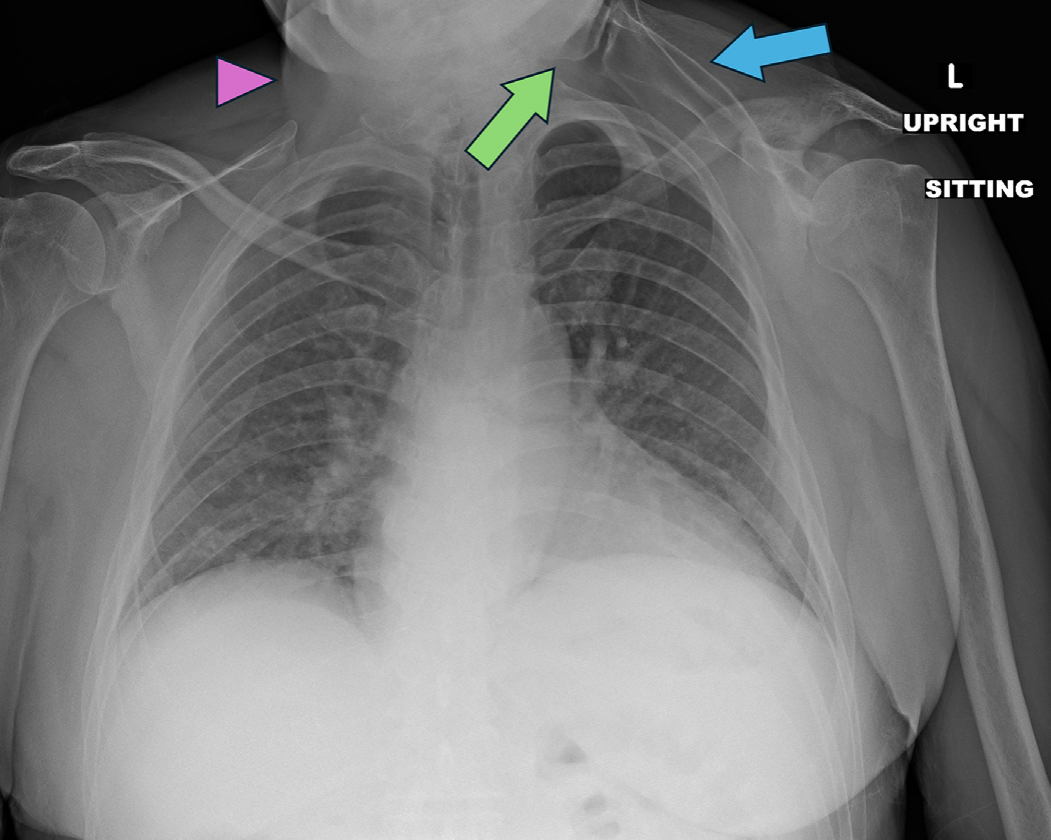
Frontal chest radiograph demonstrates a left omovertebral bone (green arrow) with scapular pseudo-articulation and left scapular elevation (blue arrow). There is suggestion of a right neck mass (pink arrowhead).
